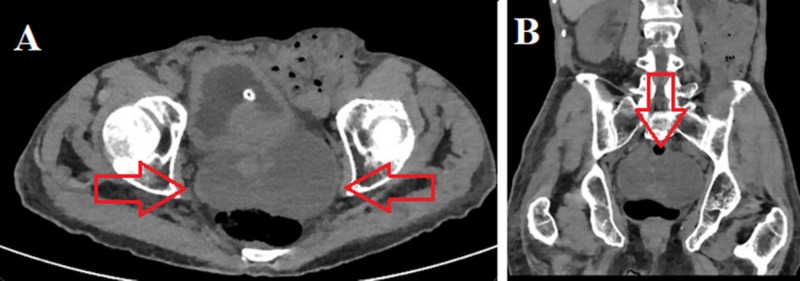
Non-contrast CT scan images, axial and coronal cuts Non-contrast CT scan images showing bilateral, large 8-cm seminal vesicle cysts (red arrows)
